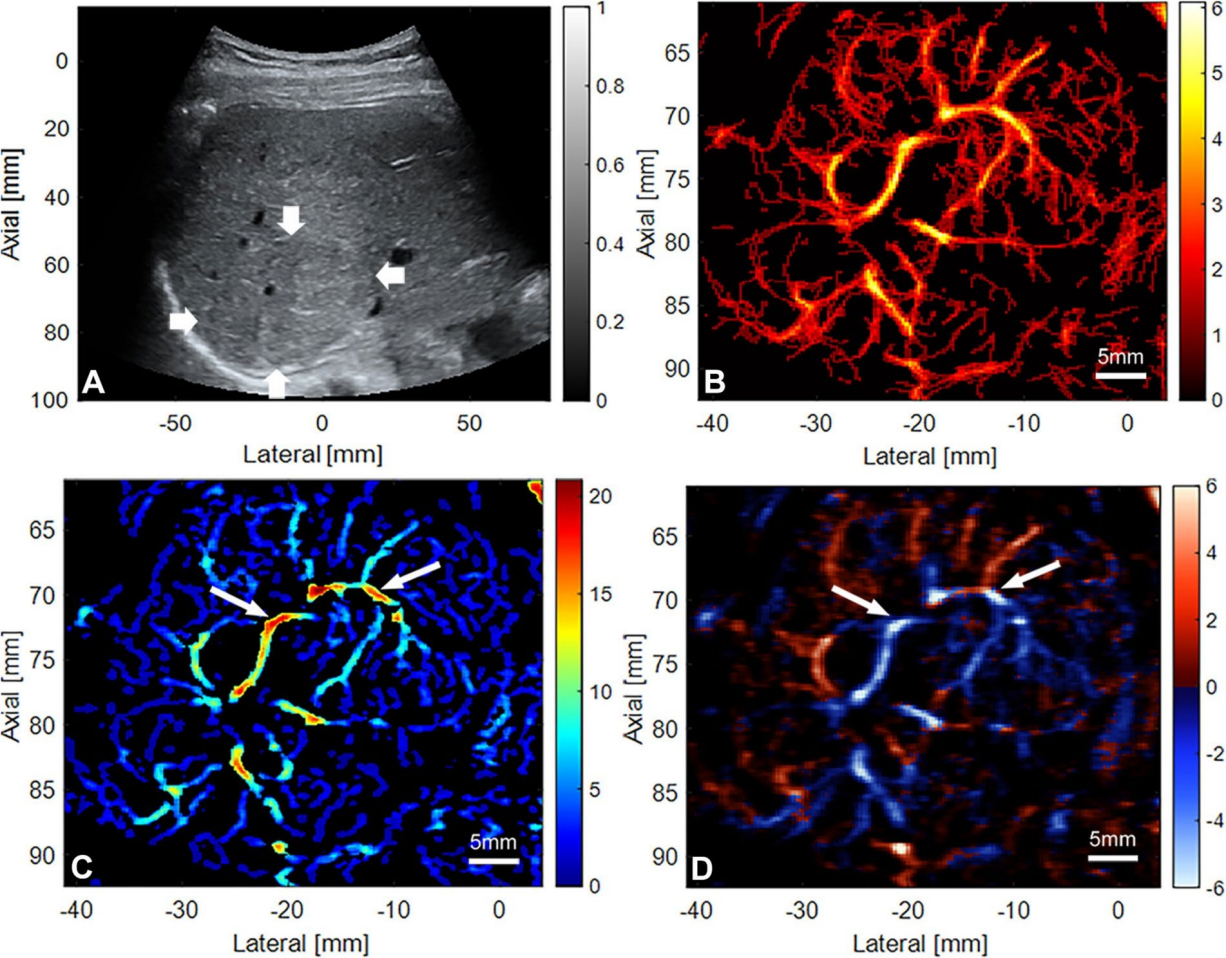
The super-resolution ultrasound imaging of a focal nodular hyperplasia case in a 38-year-old woman. A, B-mode ultrasonic image of the mass; B, the density map shows abundant microvessels within the nodule; C, the blood flow velocity map indicates that the velocity at the center of the nodule is higher than that at the periphery (white arrows), with redder colors representing higher flow velocities; D, the direction map reveals a radial blood flow pattern with varying direction, red and blue indicate flow toward and away from the transducer, respectively). (Reprineted from Zeng et al. [56])
